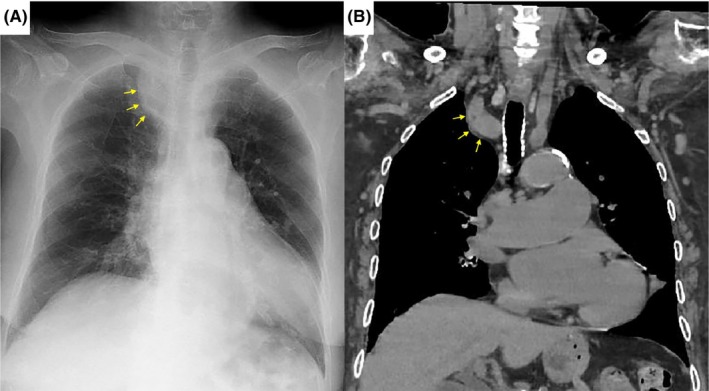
(A) Chest x‐ray demonstrating a mass‐like density in the right apex (arrows) resembling a mediastinal tumor but containing findings that are clues to the correct diagnosis: a positive extrapleural sign, negative tracheal deviation, and smooth margins. (B) Computed tomography (coronal image) revealing that the mediastinal shadow was in fact buckling of the brachiocephalic artery.
